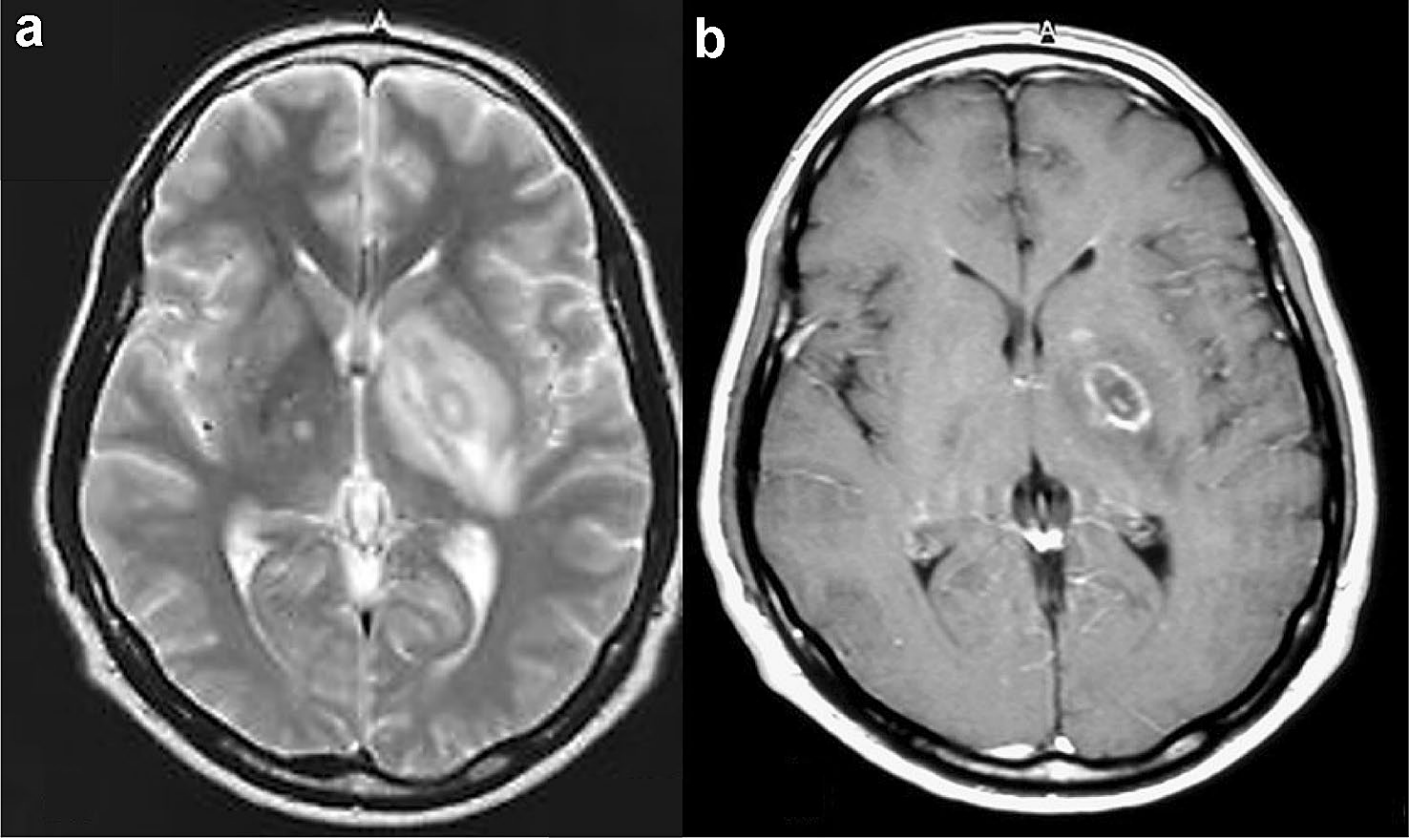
Target sign on T2-weighted image and eccentric target sign on contrast-enhanced T1-weighted image in a patient with HIV-related CNS toxoplasmosis. A mass lesion with surrounding edema was observed in the left lenticular nucleus. On T2-weighted image (a), the mass shows a concentric hyper- and hypointense zones with perilesional edema. On contrast-enhanced T1-weighted image (b), the mass shows a ring-shaped zone of peripheral contrast enhancement with a small eccentric nodule along the wall. Image b is reprinted from [9] with permission
